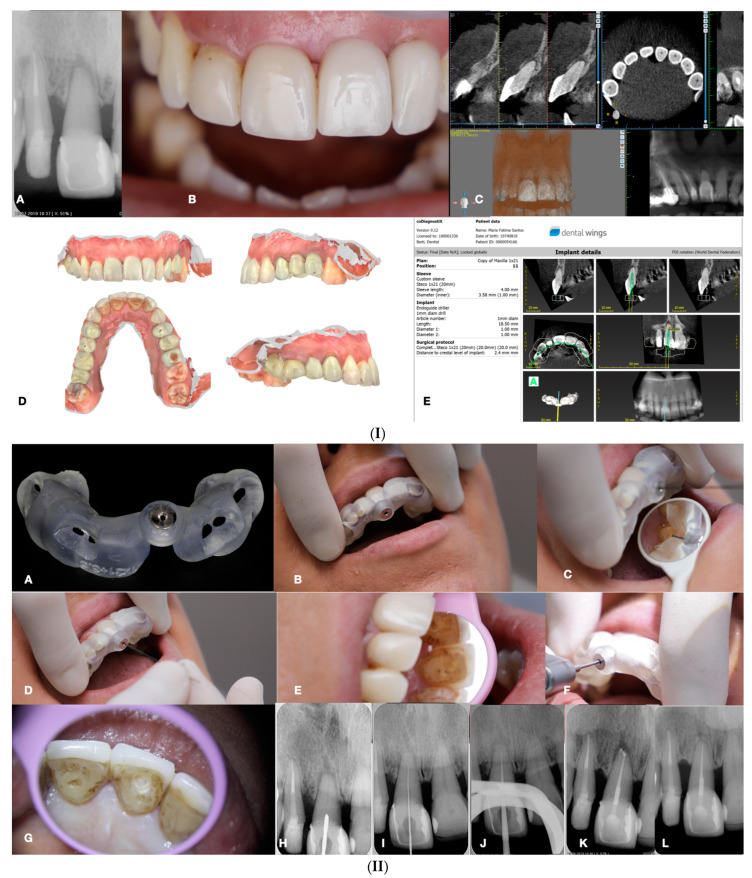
(I) (A) Preoperative periapical radiograph of calcified maxillary central incisor diagnoses with apical periodontitis. (B) Preoperative clinical buccal view of the maxillary central incisors. (C) Preoperative cbct scanning of the maxilla. (D) Preoperative intraoral scanning of the maxilla. (E) Matching the CBCT scanning with the intraoral scanning and designing the guide in the codiagnostiX 10.5 software. (II) (A) Three-dimensional printed surgical guide with lateral windows to check the adaptation. (B) Intraoral fitting of the surgical guide. (C,D) Pencil marking of the access location point lingual and buccal view. (E) Access point mark to remove the enamel. (F) Fitting of the surgical guide and drilling the initial pilot hole. (G) Clinical palatal view of the guided access. (H) Intraoperative radiograph of the pilot drill (Steko bur). (I,J) Length determination and gutta-percha fitting radiographs. (K) Postoperative radiograph. (L) Two-year follow-up radiograph reveals healing (courtesy of Dr. Hugo Sousa Dias).
